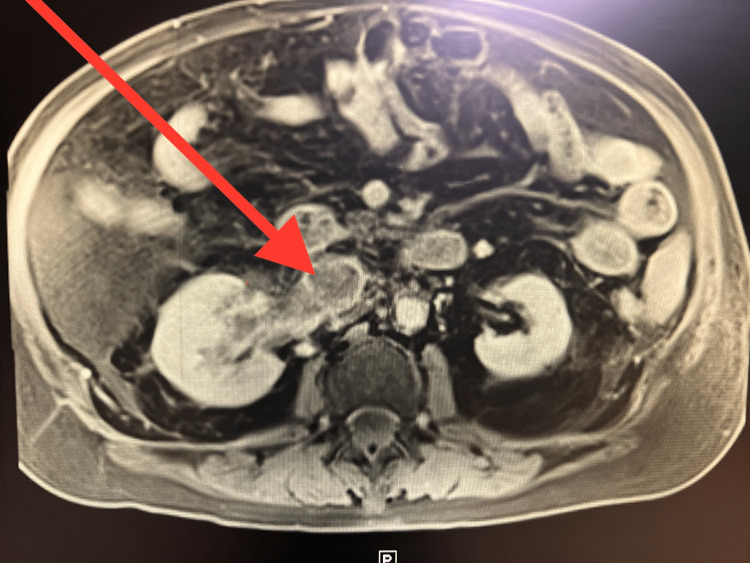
MRI abdomen revealing a filling defect within the right renal vein.
